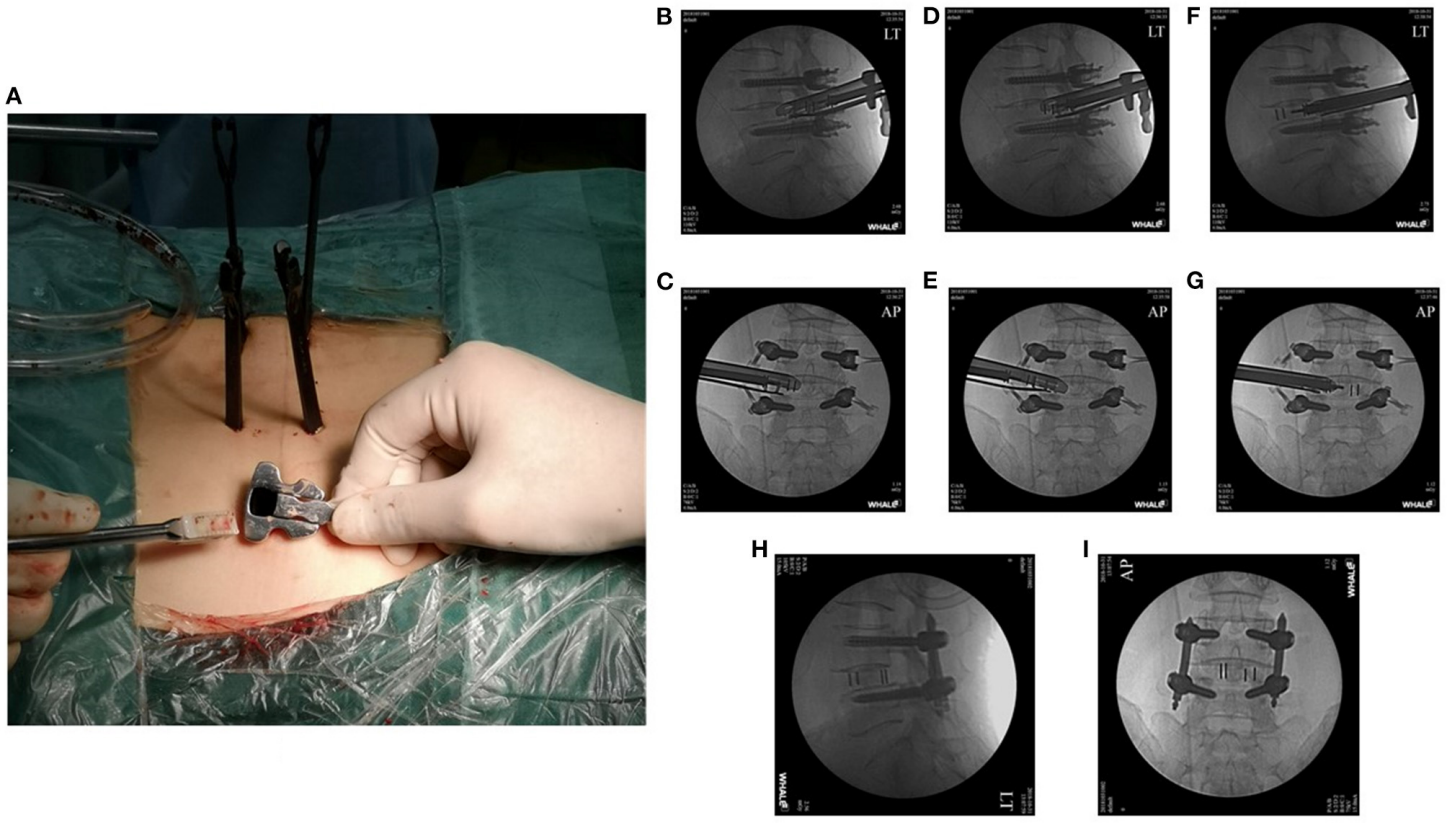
A conventional cage was placed through the working tube under fluoroscopic guidance (A–G). The final cage position is shown in the anteroposterior and lateral X-rays (H,I).
